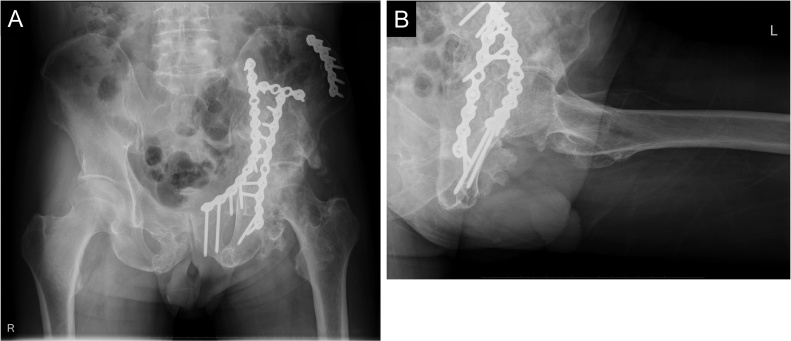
(A) Anteroposterior and (B) lateral pelvic radiographs at the final follow-up, 5.5 years after HO resection. The patient developed no HO recurrence.
